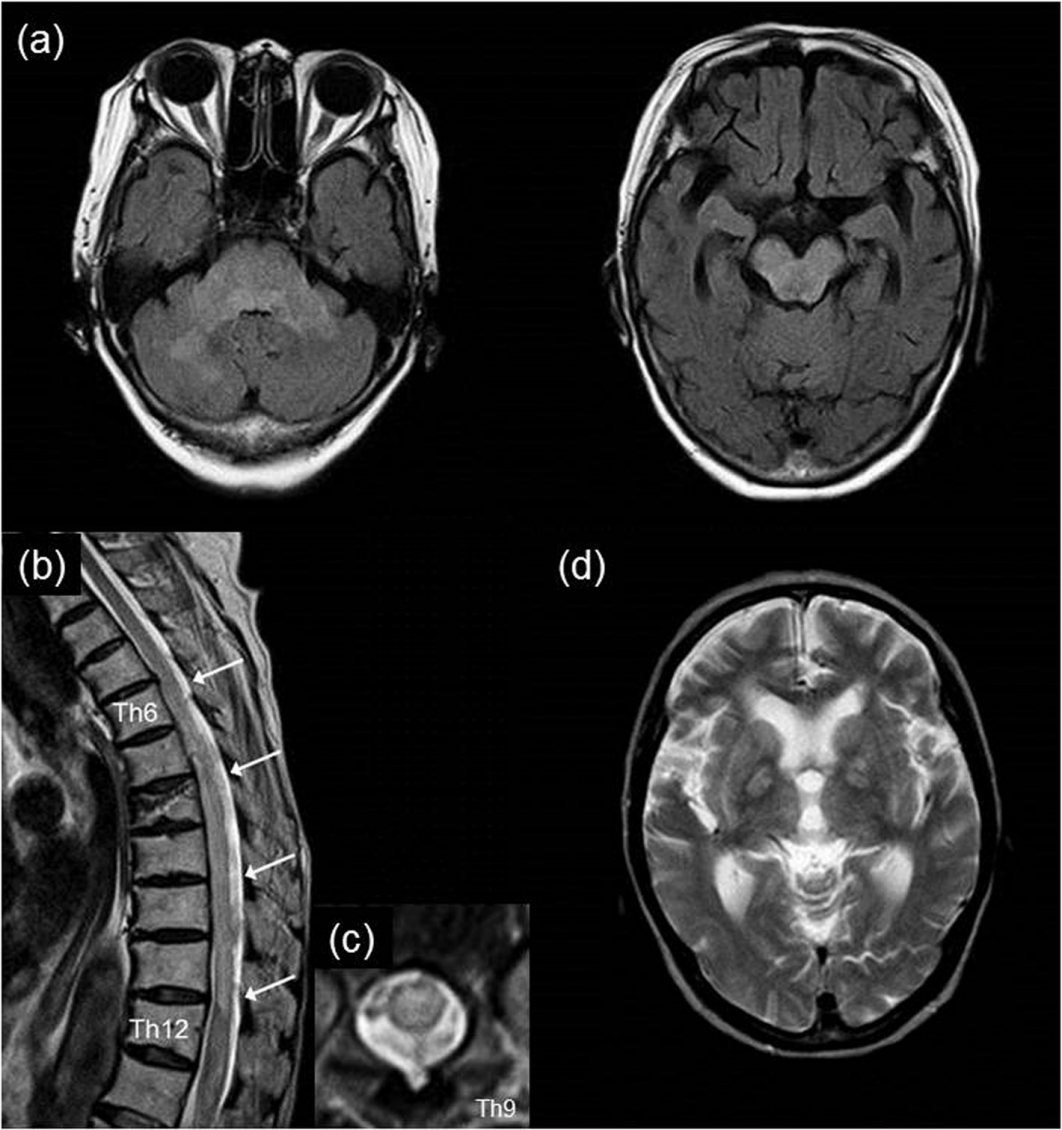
Brain magnetic resonance imaging performed on admission. Axial fluid-attenuated inversion recovery images showed bilateral symmetric hyperintensity in the brainstem and cerebellum (a). Magnetic resonance imaging of the spinal cord performed on day 11. Sagittal T2-weighted image revealed an intramedullary lesion from the level of the 6th to the 12th thoracic vertebrae (b, arrows). There is compression fracture in the 8th thoracic vertebra. Axial T2-weighted image at 9th thoracic vertebrae indicated transverse myelitis (c). T2-weighted magnetic resonance imaging on day 19 showed bilateral symmetric high-intensity lesions in the globus pallidus (d). Abbreviation: Th, thoracic.
